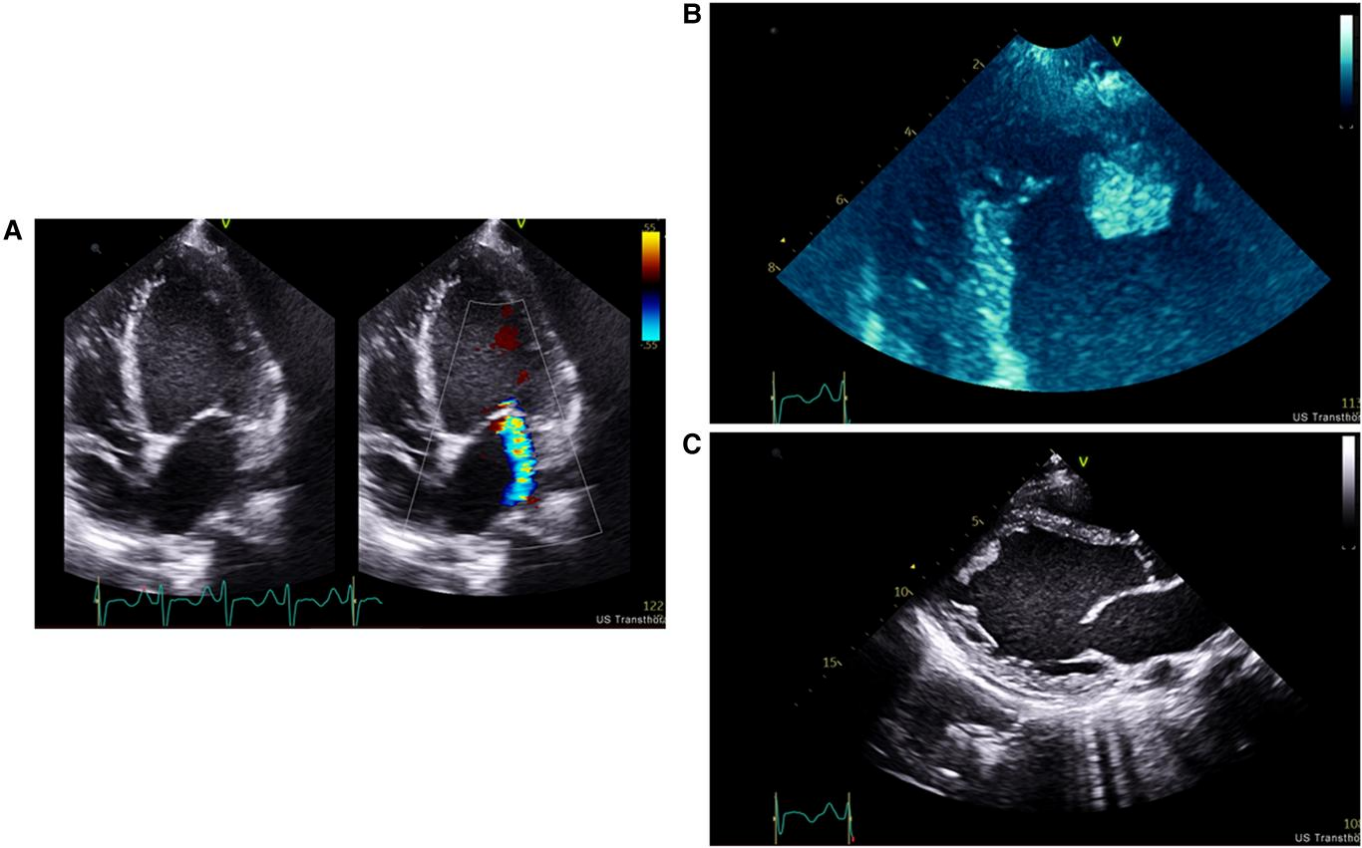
Transthoracic echocardiogram on admission. (*A*) Apical four-chamber view with colour flow Doppler at the level of the mitral valve. (*B*) Apical four-chamber view focused on the left ventricle apex with intra-cardiac thrombus. (*C*) Parasternal long-axis view.
